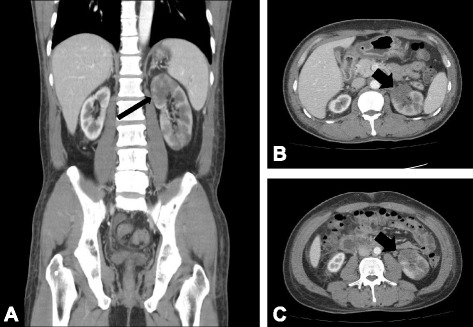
Contrast-enhanced abdominal computed tomography. a Coronal and (b, c) transverse scans showed left kidney enlargement with a multifocal infarcted area (*arrows*). Neither renal artery was traced from the proximal part on computed tomography
